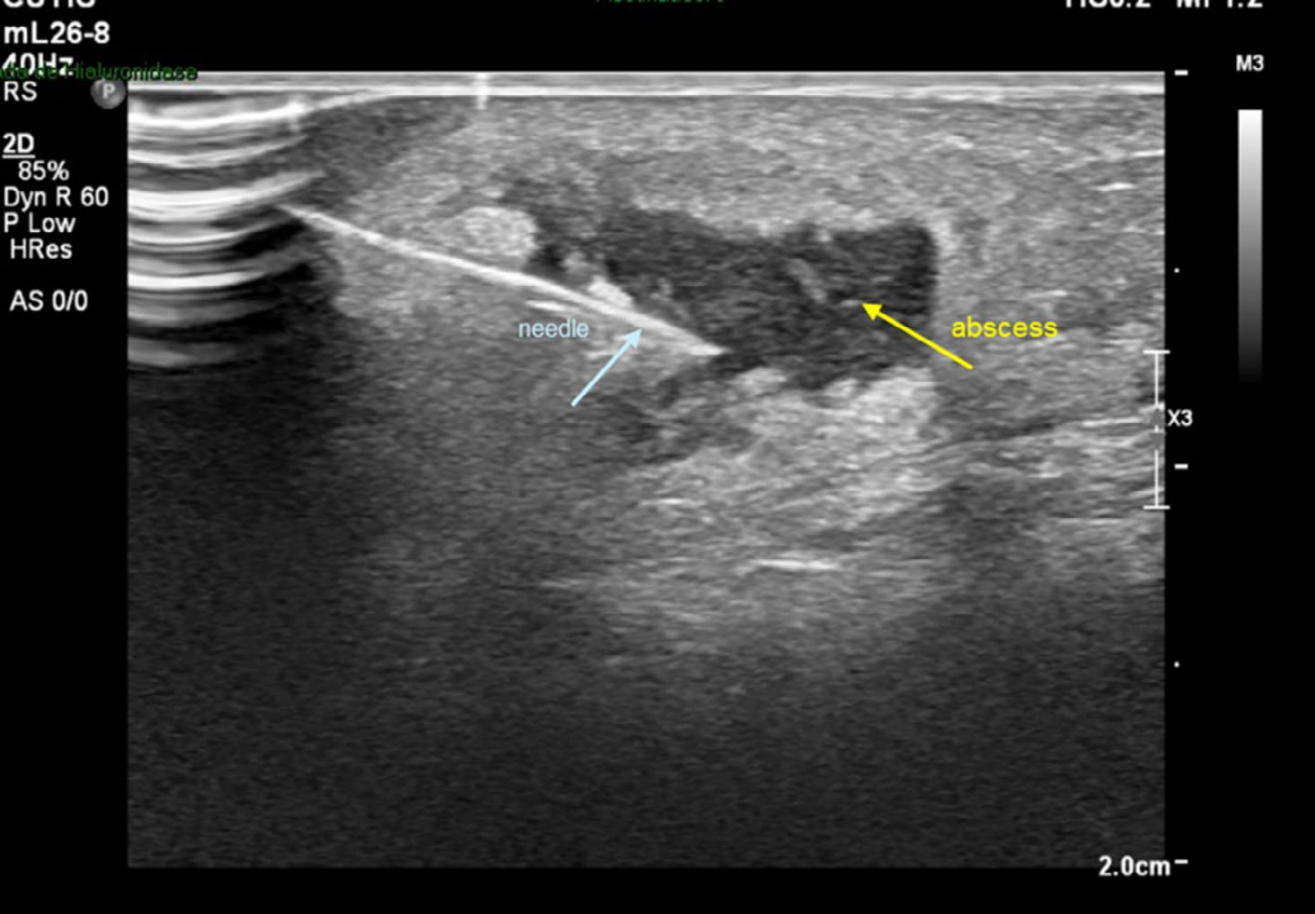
Ultrasound image demonstrating the needle inside the abscess during the mMCP.
